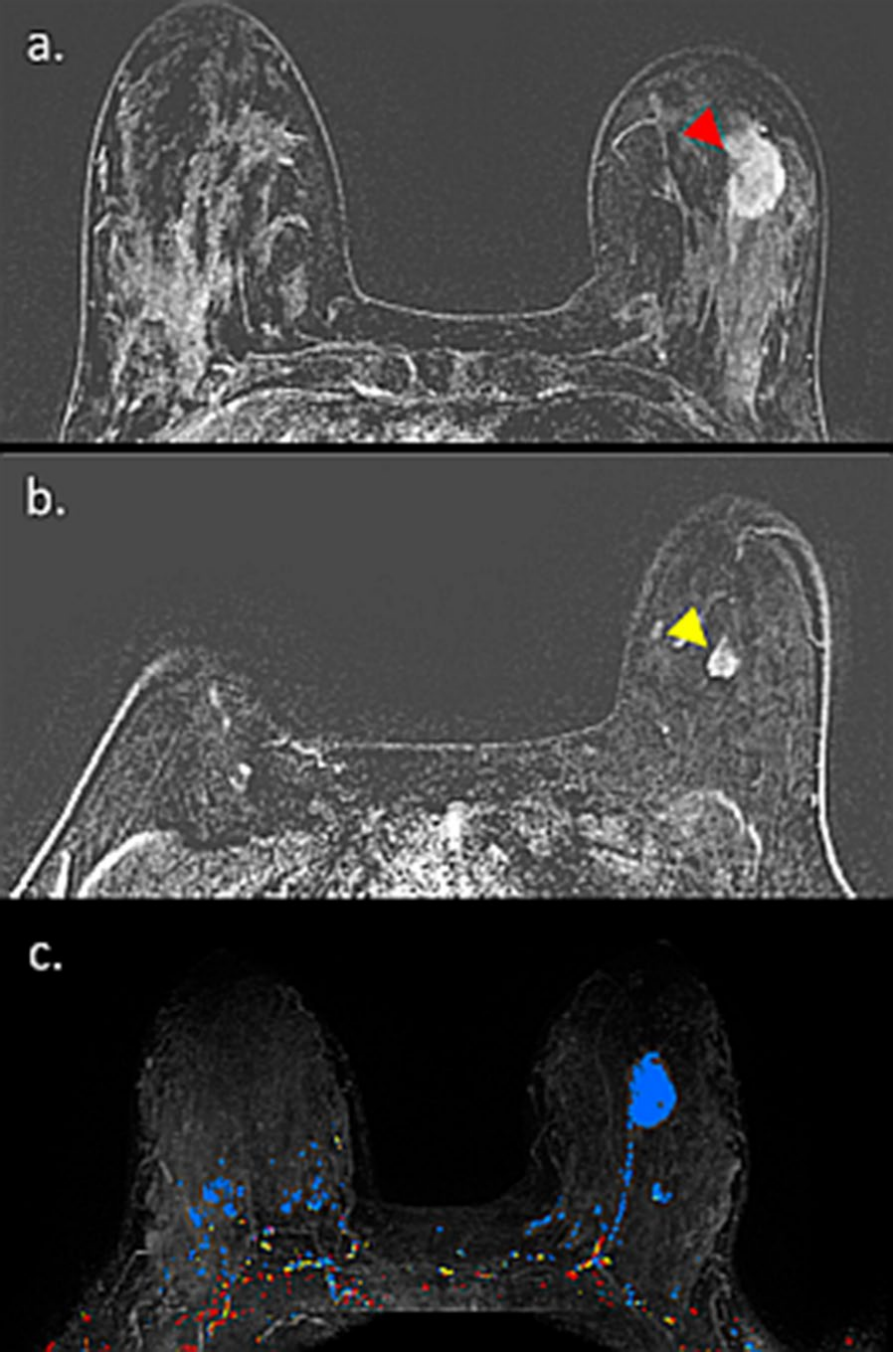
MRI of a complicated case of IDC with lactation changes. Axial subtracted DCE-MRI and CAD of lactating patient with newly diagnosed PABC, undergoing preoperative evaluation are shown. The tumor, 2.8 cm IDC in the left breast, is well visible on top of the surrounding BPE (a). Yet, additional ipsi-lateral enhancing 0.9 cm lesion is apparent (b). Upon focused US and US-guided biopsy, the second lesion came up to be adenosis and lactating changes on pathology. Interestingly, CAD depicted both lesions, as well as slight BPE in blue color, which corresponds to persistence wash-in pattern which usually represents a benign pattern (c)
